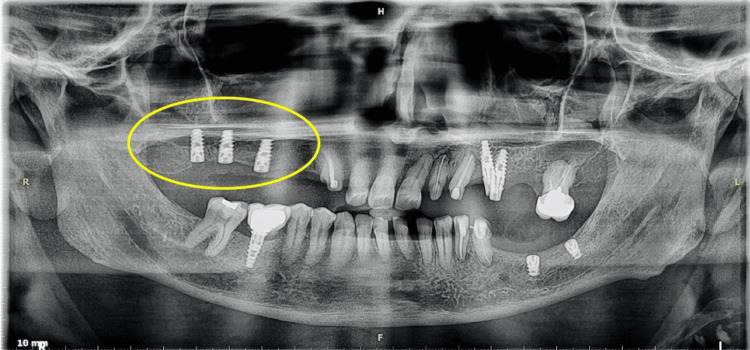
Postoperative OPG A three-implant prosthesis was placed with the help of Densah burs on the marked region. OPG, orthopantomogram
